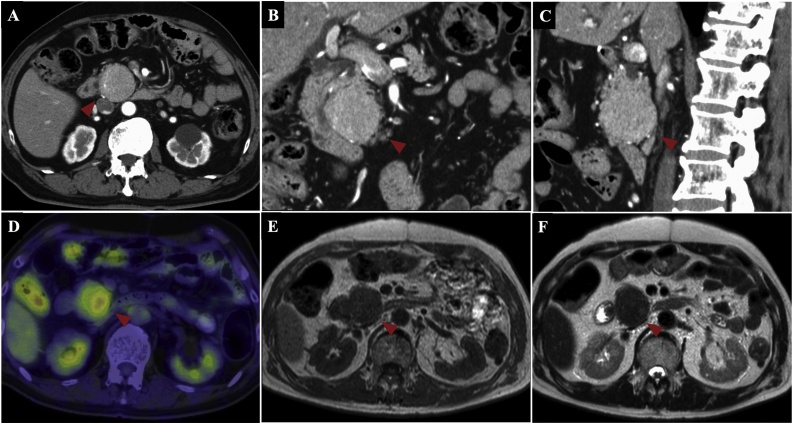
Contrast-enhanced computed tomography reveals a 40-mm well-defined solid mass in the pancreatic head (A, B: Coronal section, C: Sagittal section). Arrowheads indicate the tumor. The solid mass demonstrates high fluorodeoxyglucose uptake on positron emission tomography (D). On both T1- and T2-weighted magnetic resonance imaging, the mass is marginated with a low intensity. There is no apparent invasion of other organs (E, F).
